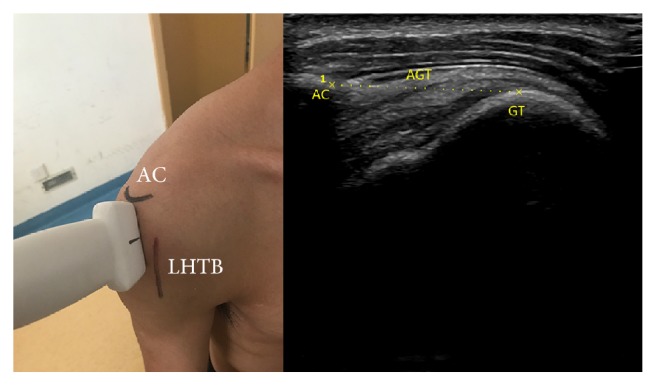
Anatomical structures: AC (acromion); LHTB (long head tendon of biceps); the position and orientation of transducer; and the ultrasound image: GT (greater tuberosity) and AGT (acromion-greater tuberosity).
